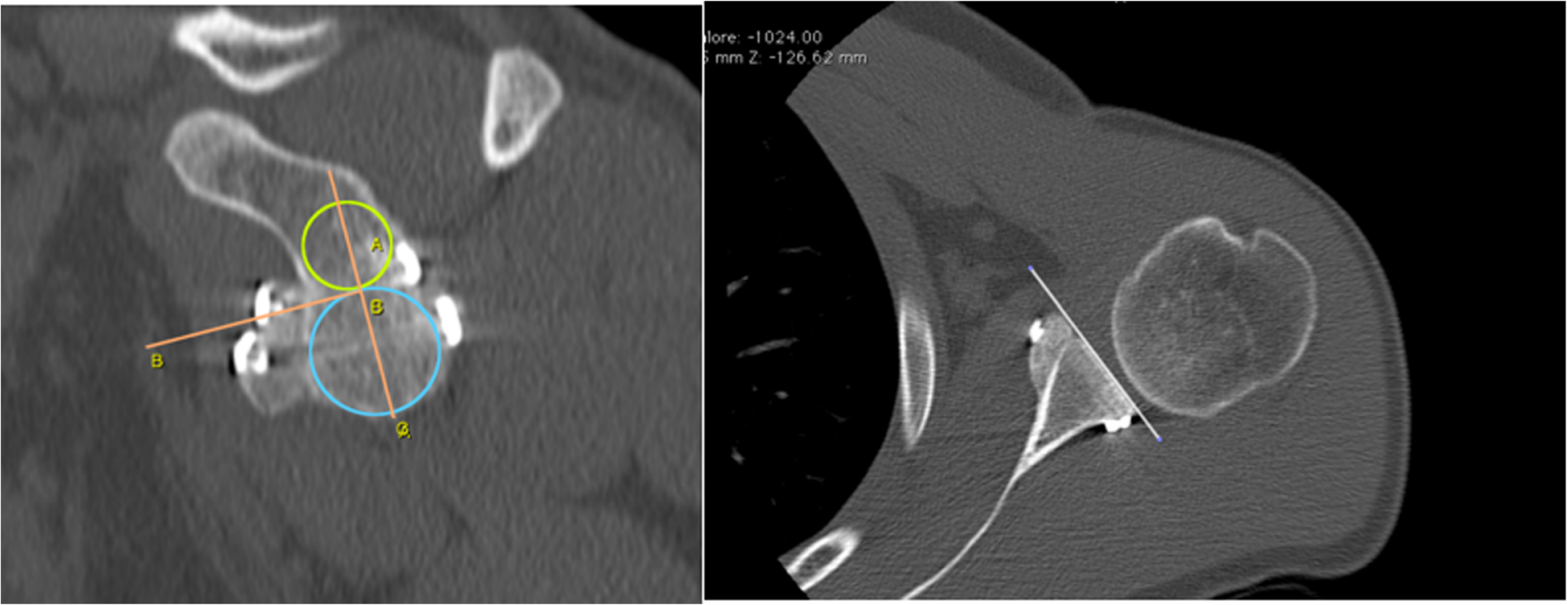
Computed tomography images showing bone graft healing and remodeling after one year
